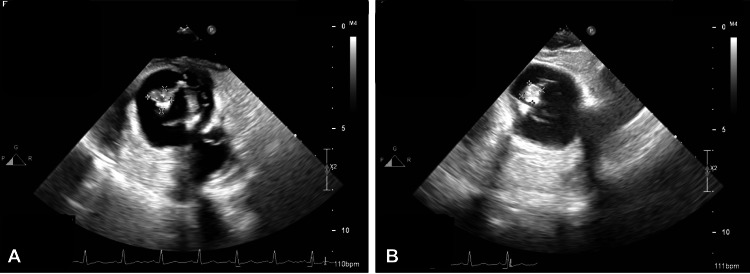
Transesophageal echocardiogram Image A: vegetation on the prosthetic valve; Image B: abscess in the mitral-aortic continuity
